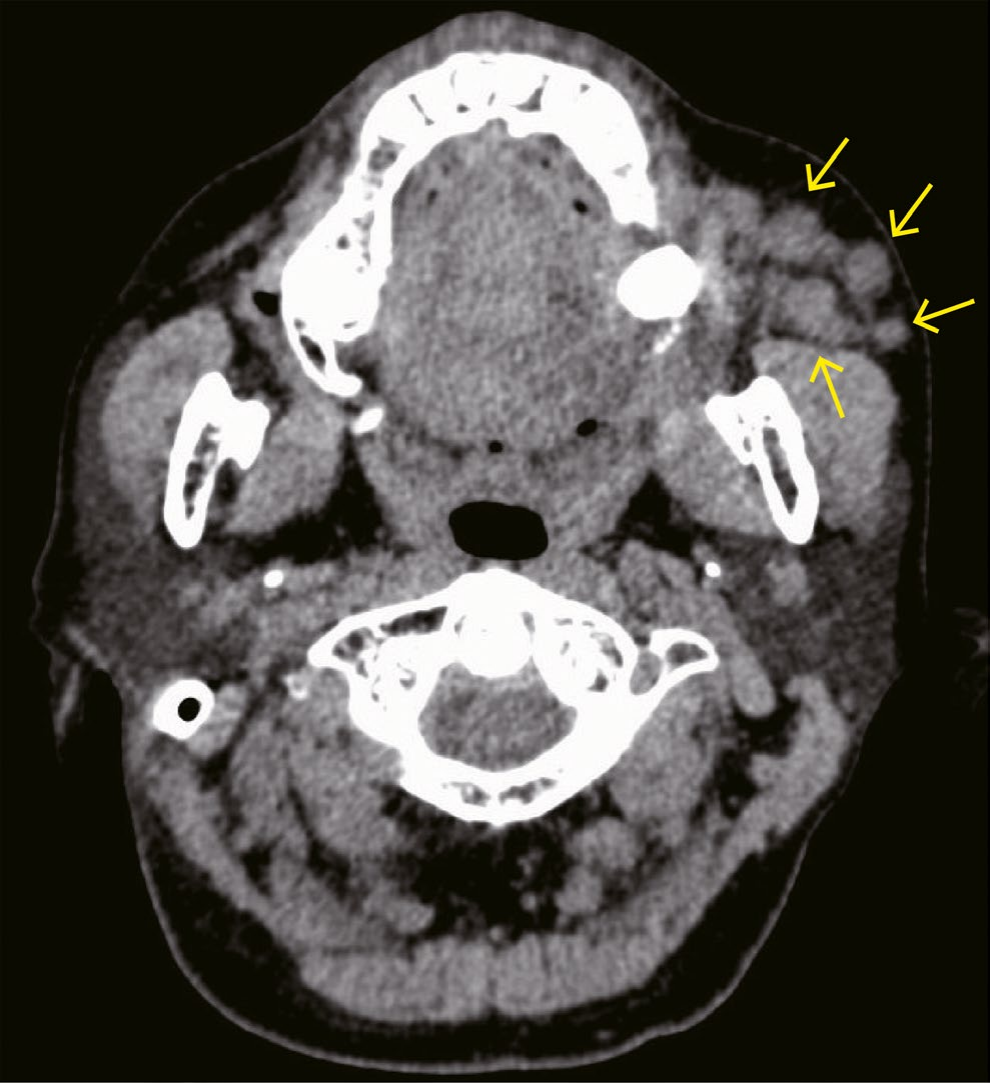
Preoperative nonenhanced CT image. Under the left cheek, some irregular tumor masses were observed (arrows). CT, computed tomography
